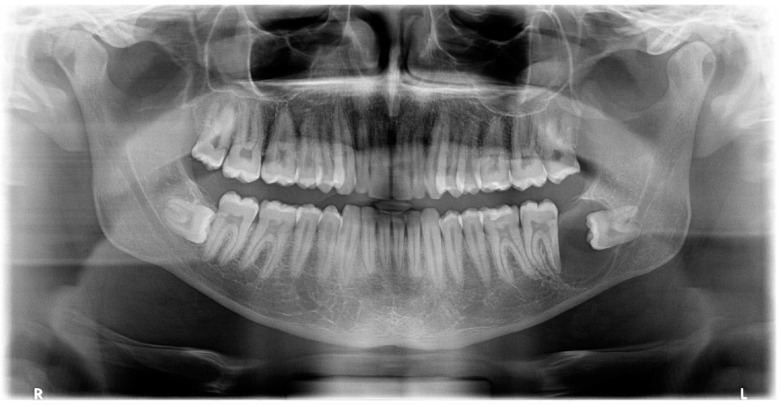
Panoramic X-ray of case #1.
